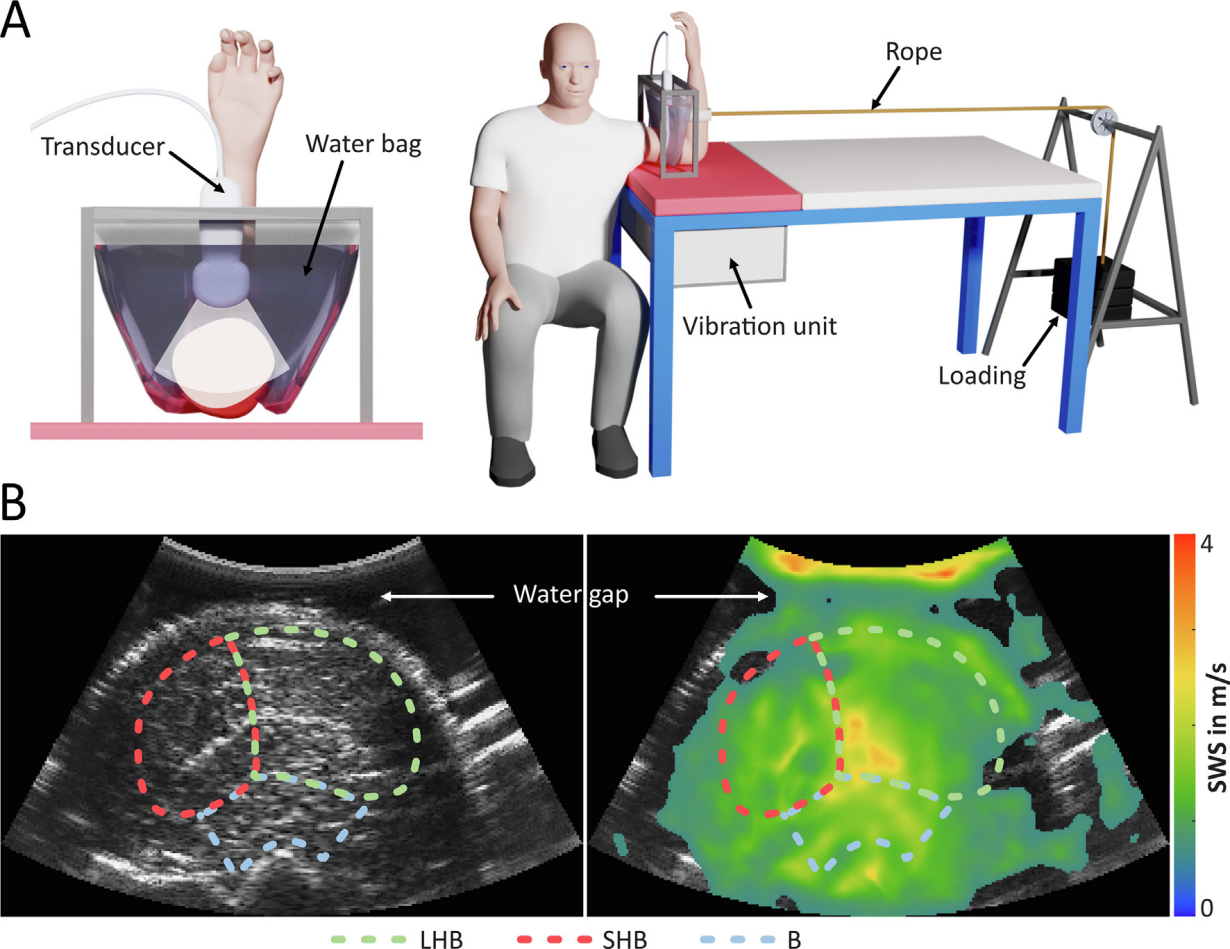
A: Setup of time-harmonic elastography (THE) for the loading phase. Left: The transducer is coupled to the upper arm with a water bag. This avoids external pressure on the muscle and extends the ultrasonic field-of-view. The elbow support for the pre- and postload phases and the transducer stand to avoid unintentional changes of the image section are not shown. Right: Position of the subject on the patient bed with the integrated vibration unit and the cable with the loading weight; B: B-mode image (left) and shear-wave speed (SWS) map (right) of the transverse view of the upper arm with corresponding region of interest (LHB – long head of biceps brachii, SHB – short head of biceps brachii, B – brachialis muscle).
